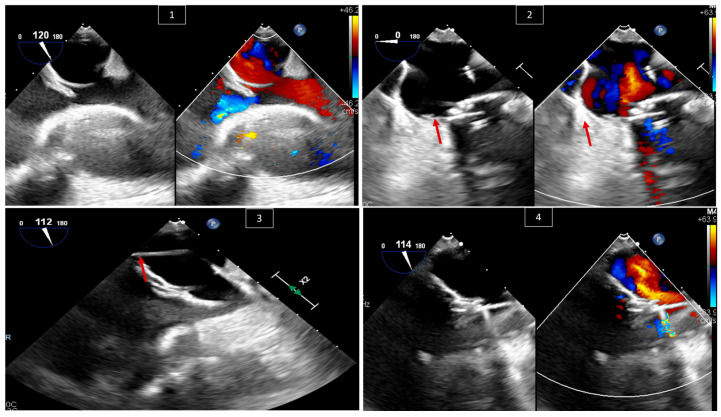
Guiding the percutaneous closure of the SSVD of a 70-year-old female. (1) The SVC right atrium (RA) and the sinus venosus defect seen with and without color Doppler with right upper anomalous pulmonary vein (RUPV) return in red at 120 degrees. (2) The same defect at 0 degrees with the wire (arrow) in the SVC and confirming its position in the RA and not through the defect. (3) Confirming the position of the wire (arrow) from the interatrial septum and in the right upper pulmonary vein (the anomalous vein) to check pressures during the intervention. (4) Post implantation of the covered stent in the SVC showing closure of the SSVD with no obstruction to pulmonary vein flow (in red).
